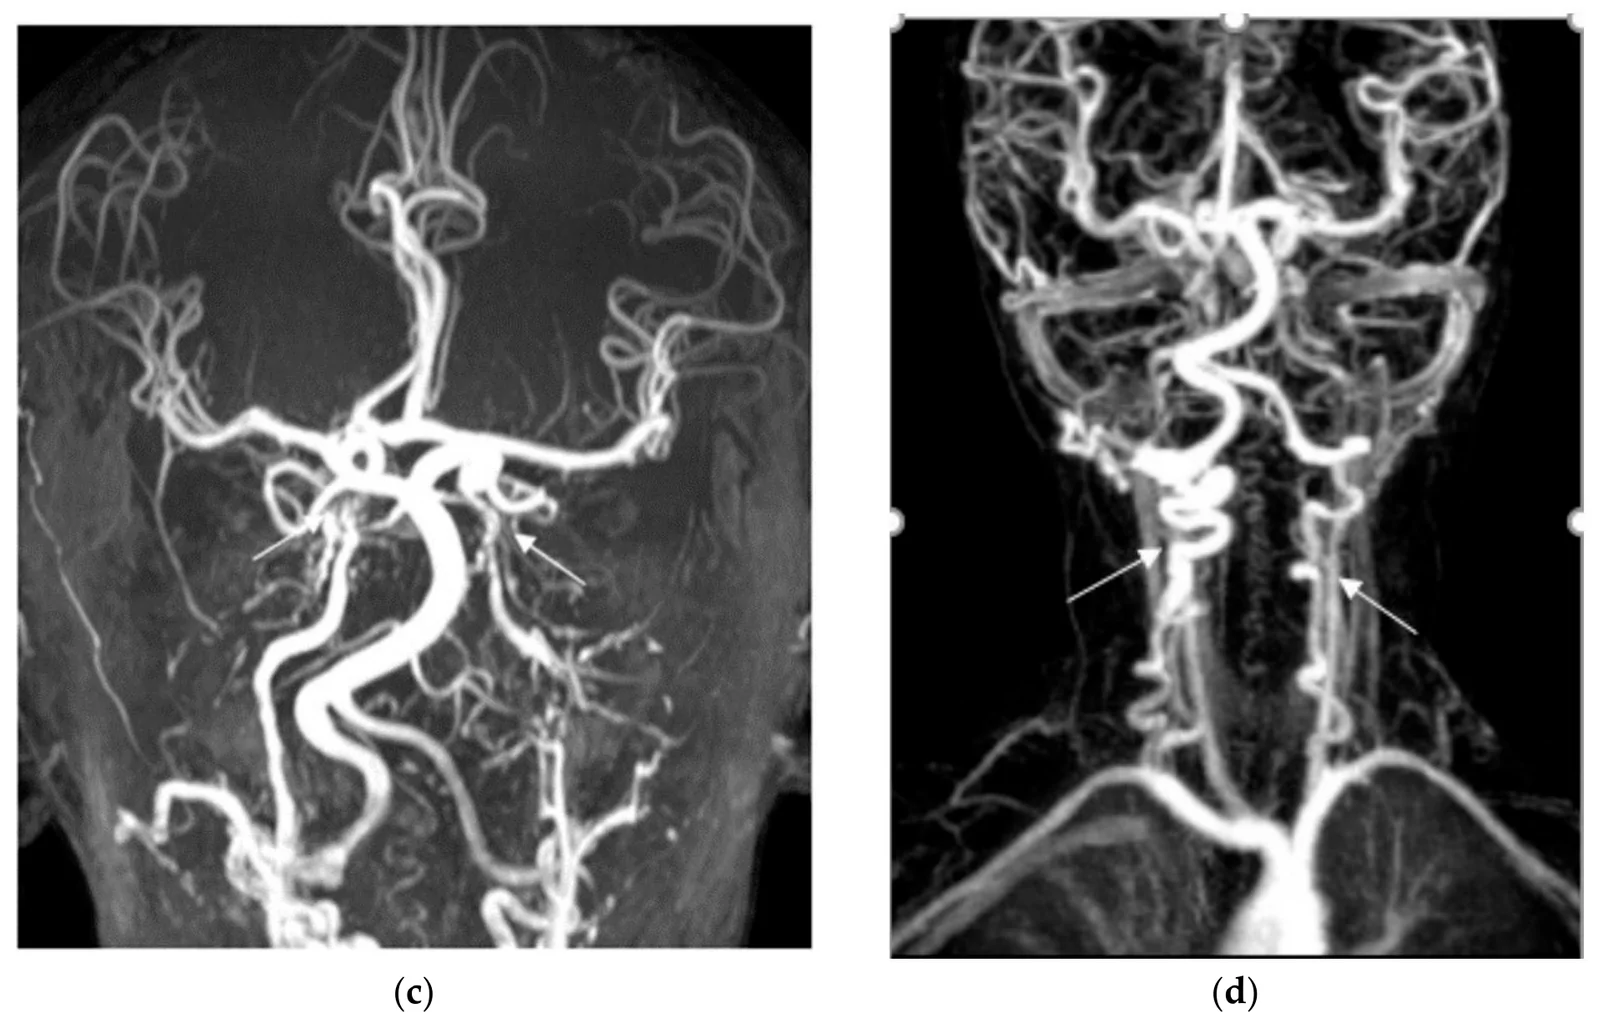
Neuroimaging findings in Case 2: an 8-year-old girl with chronic bilateral watershed infarctions due to bilateral internal carotid artery occlusion in Takayasu arteritis. (a) Axial FLAIR image demonstrates chronic watershed infarcts with encephalomalacia and surrounding gliosis, more prominent in the right hemisphere, with similar gliotic changes in the contralateral hemisphere. (b) Coronal T2-weighted image demonstrates chronic watershed infarction with encephalomalacia in the right hemisphere. (c) Intracranial magnetic resonance angiography (MRA) demonstrates distal occlusion of the bilateral internal carotid arteries with collateral supply from the posterior circulation. (d) Neck magnetic resonance angiography (MRA) demonstrates tortuosity of the bilateral common carotid and internal carotid arteries with distal occlusion of the bilateral internal carotid arteries.
